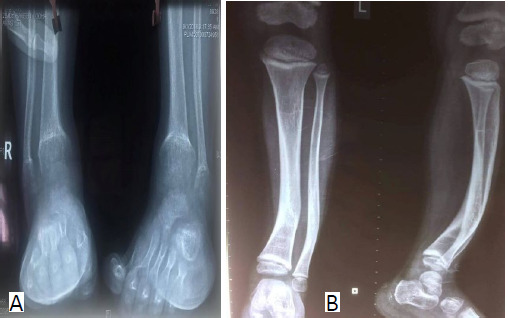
X-ray before treatment, A) Right leg and B) left leg at the time of presentation.
